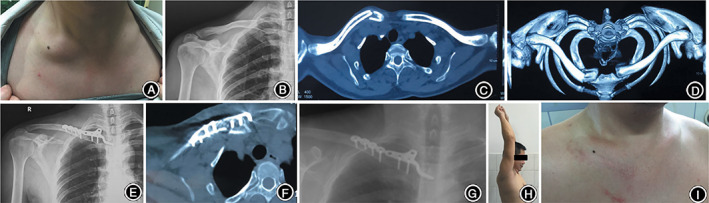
A 40‐year‐old man was injured by machine compression during work 2 months previously. He was treated conservatively at a local hospital and then presented to our institute for further intervention because of persistent pain and a dermal prominence. (A) An obvious dermal prominence was found at the medial end of the clavicle. (B) A preoperative radiograph and (C, D) CT scan showed a displaced right medial clavicle fracture with no obvious bony callus. (E, F) A postoperative radiograph and CT scan showed excellent fracture alignment and fixation with a Balser plate. (G) At 12 months postoperatively, fracture union was present with no implant displacement or breakage. (H) The patient’s full range of motion of the shoulder joint was restored with (I) no pain or dermal prominence.
